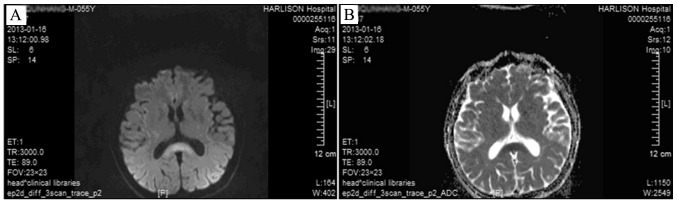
Magnetic resonance imaging showed symmetric abnormal signals in the splenium of the corpus callosum. (A) Diffusion-weighted imaging sequences showed a high signal in the splenium of the corpus callosum. (B) Apparent diffusion coefficient sequences showed a low signal in the splenium of the corpus callosum.
